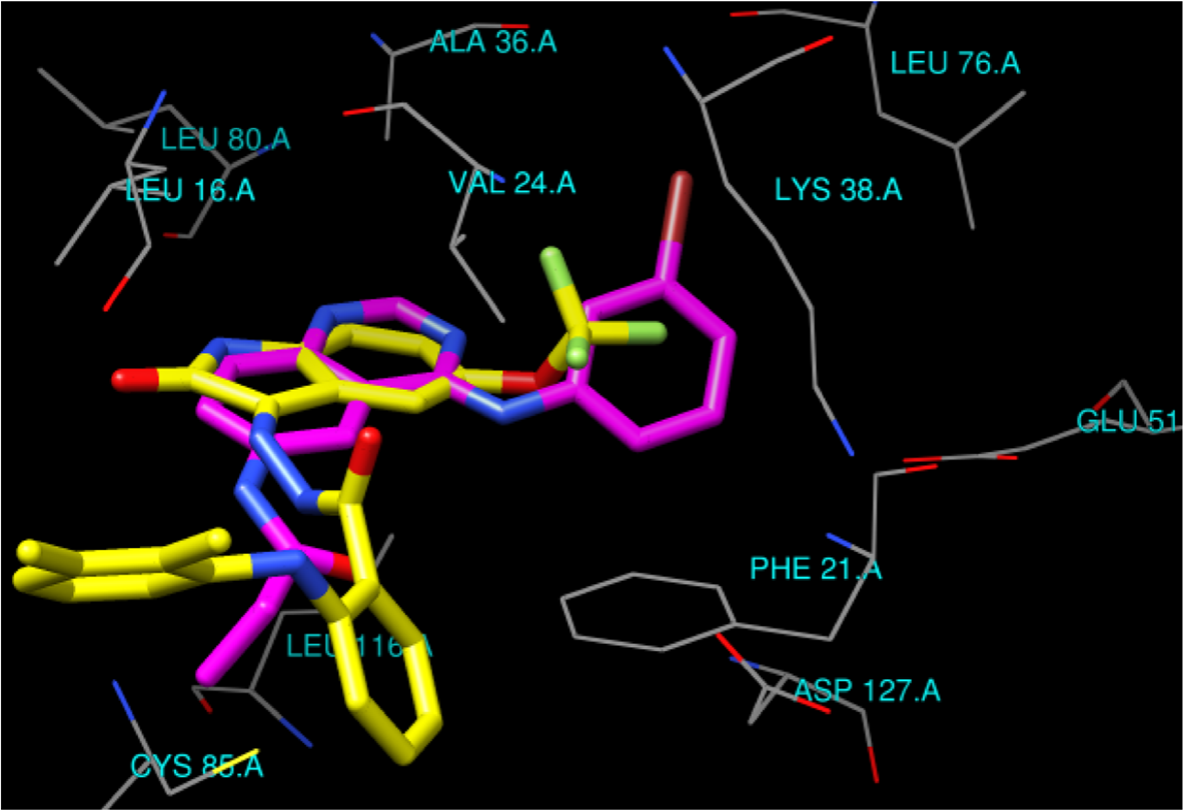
Compound H5 (colored Yellow) docked in EGFR binding site and overlaid with DJK_3021_A x-ray structure (colored magenta).
